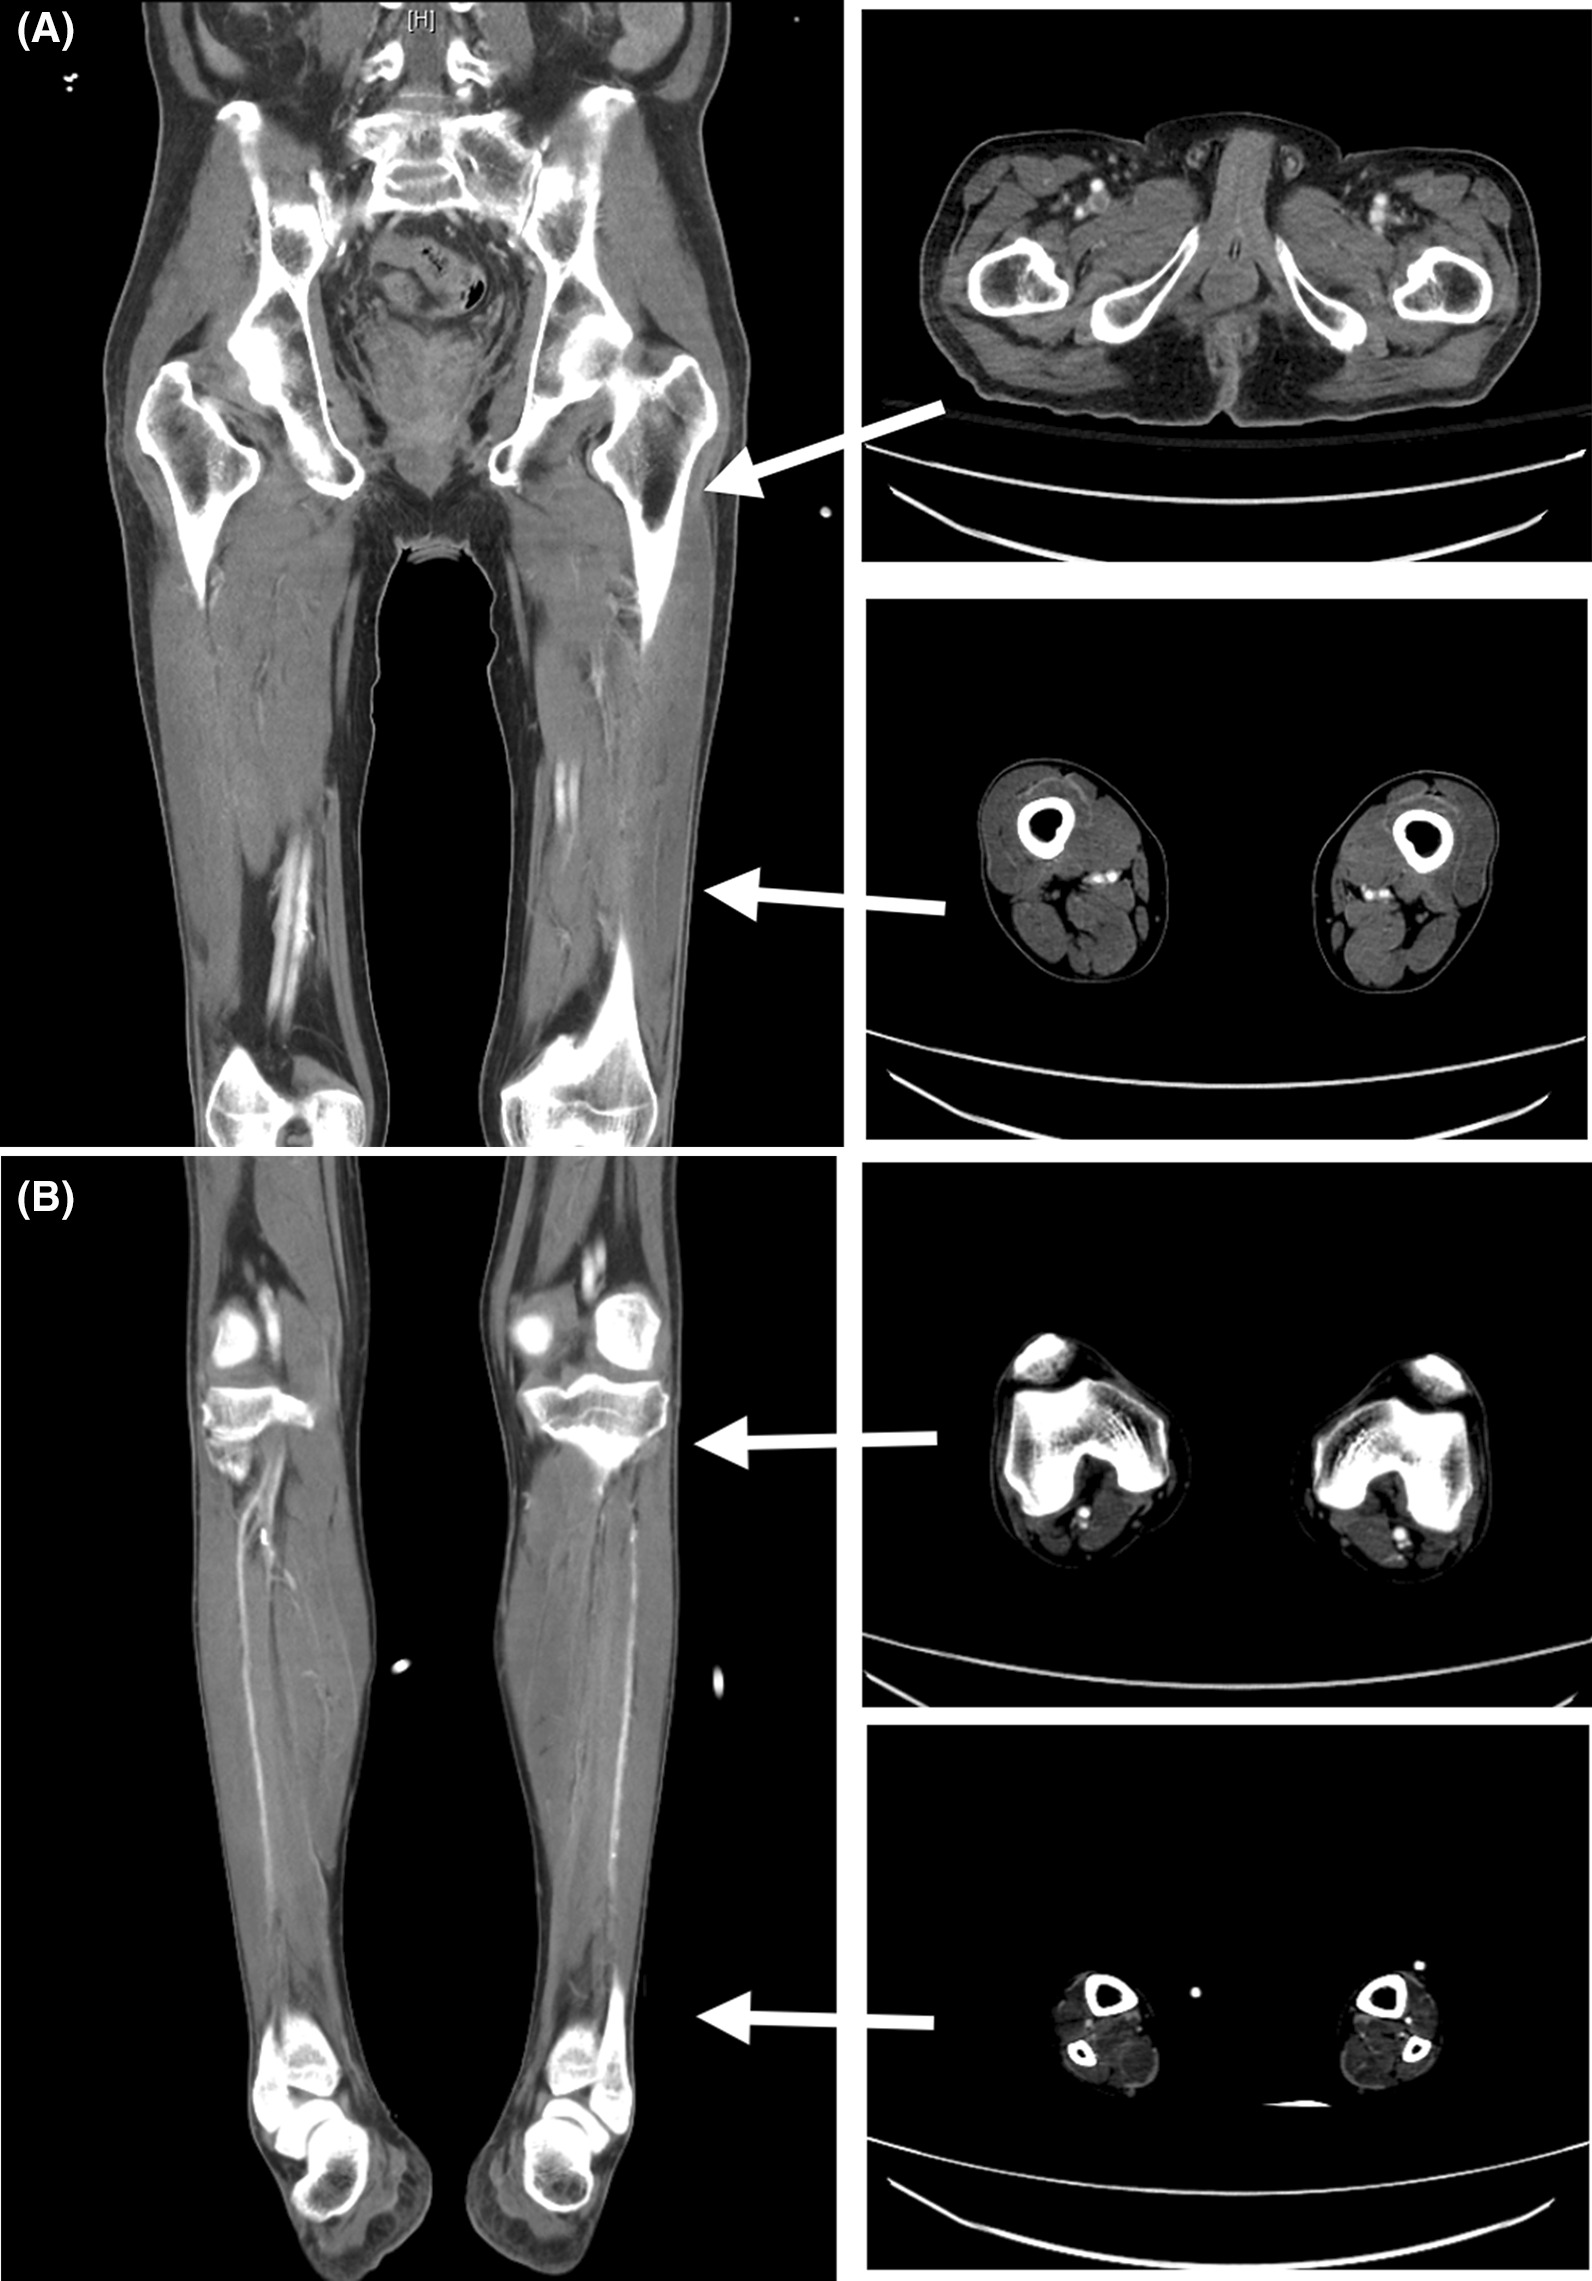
In the lower extremity CTA series, delayed artery flow was detected from the proximal leg to the distal site. A No specific embolic lesion was found from the common femoral artery to the distal superficial femoral artery. B A similar finding was documented in the distal part of the extremities: patent artery flow without occlusion in the popliteal and below knee arteries
